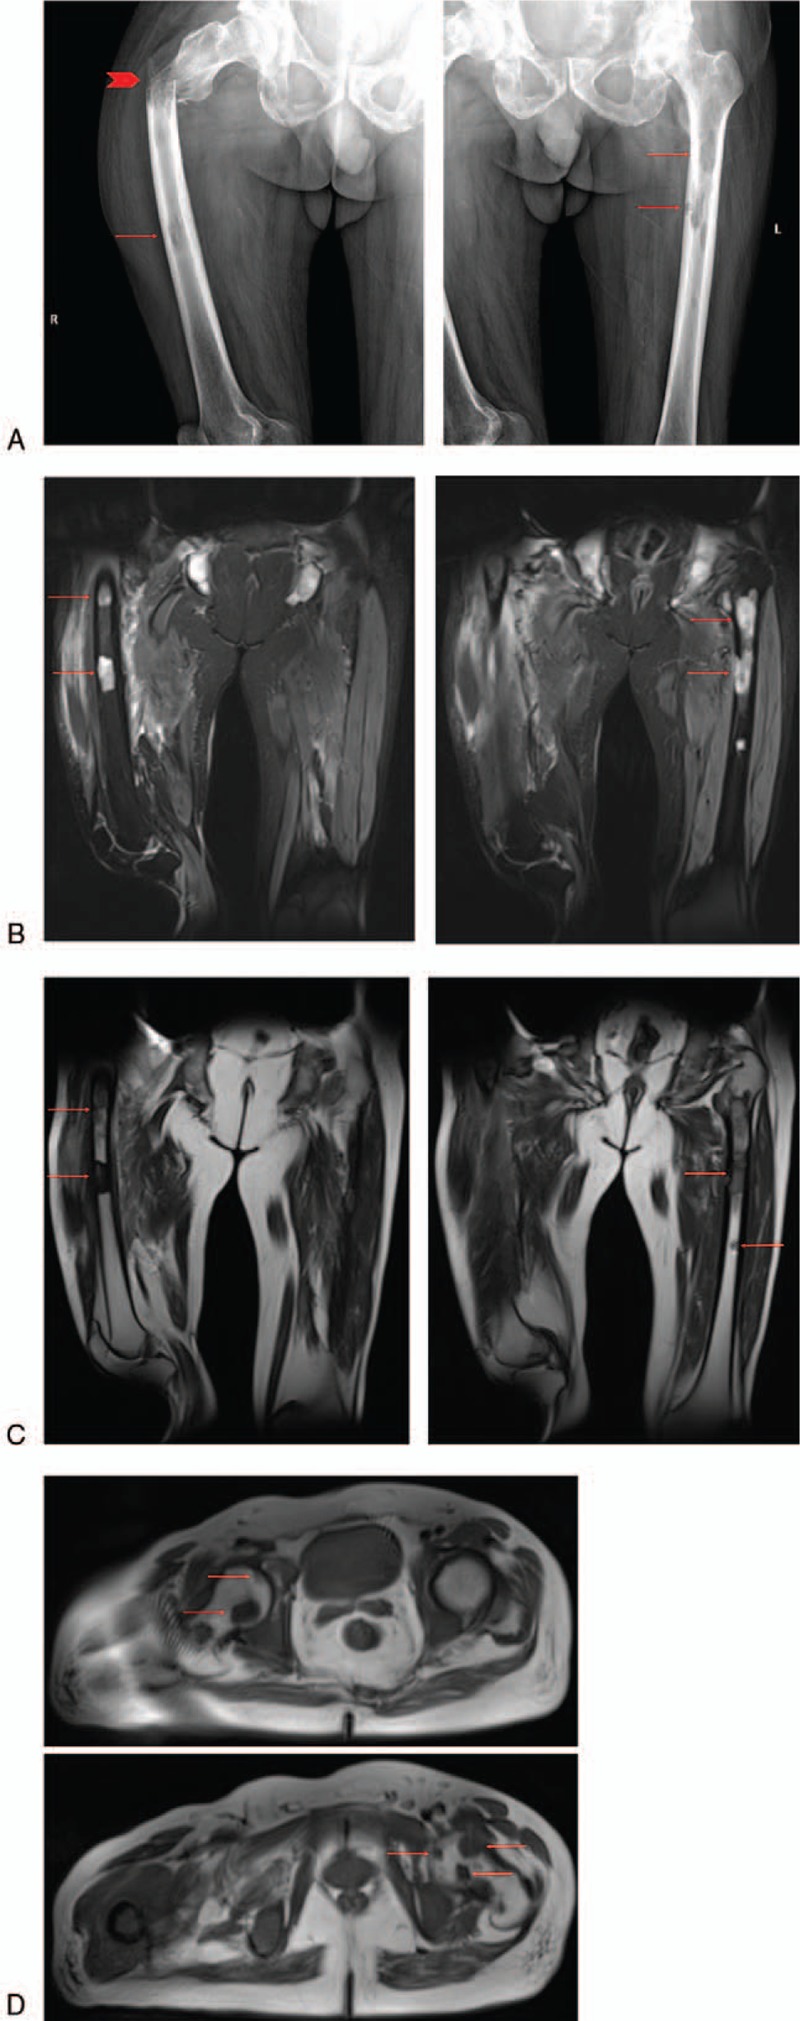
The x-ray (A) and MRI examination (B–D) of the patient's femurs, showed a pathological fracture (the arrowhead) on the right femur with multiple low-density lesions of both femurs, which were bone destruction (the arrows). MRI = magnetic resonance imaging.
